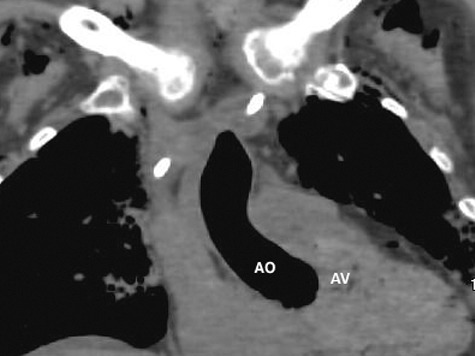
Coronal CT – lung windows – large amount of abnormal air in ascending aorta (AO). Aortic valve (AV) is delineated nicely demonstrated due to surrounding air.
